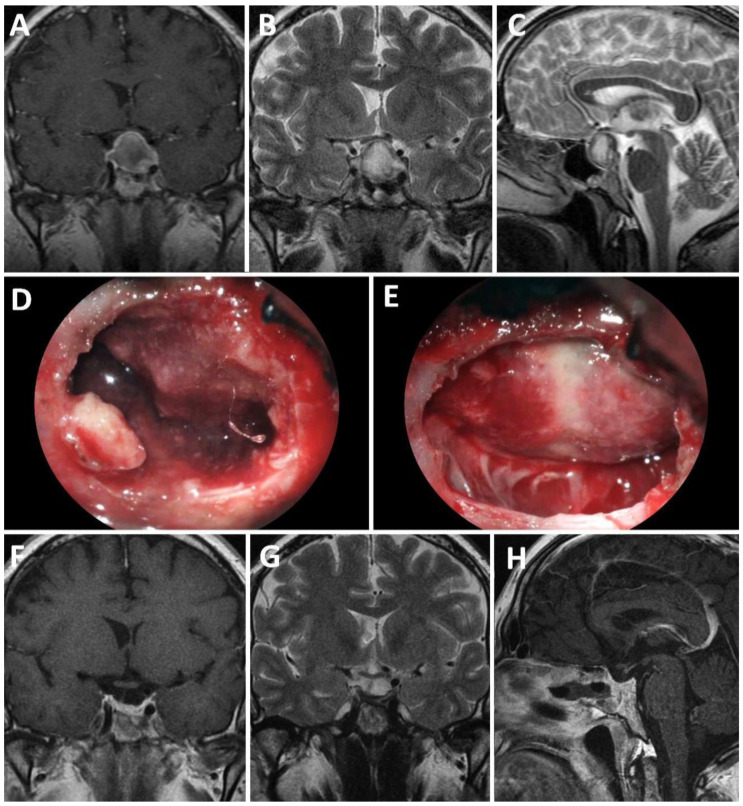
A 35-year-old patient with pituitary apoplexy in the context of a non-functioning macroadenoma. (A–C): Preoperative MRI; (D,E): intraoperative findings; (F–H): postoperative MRI.
